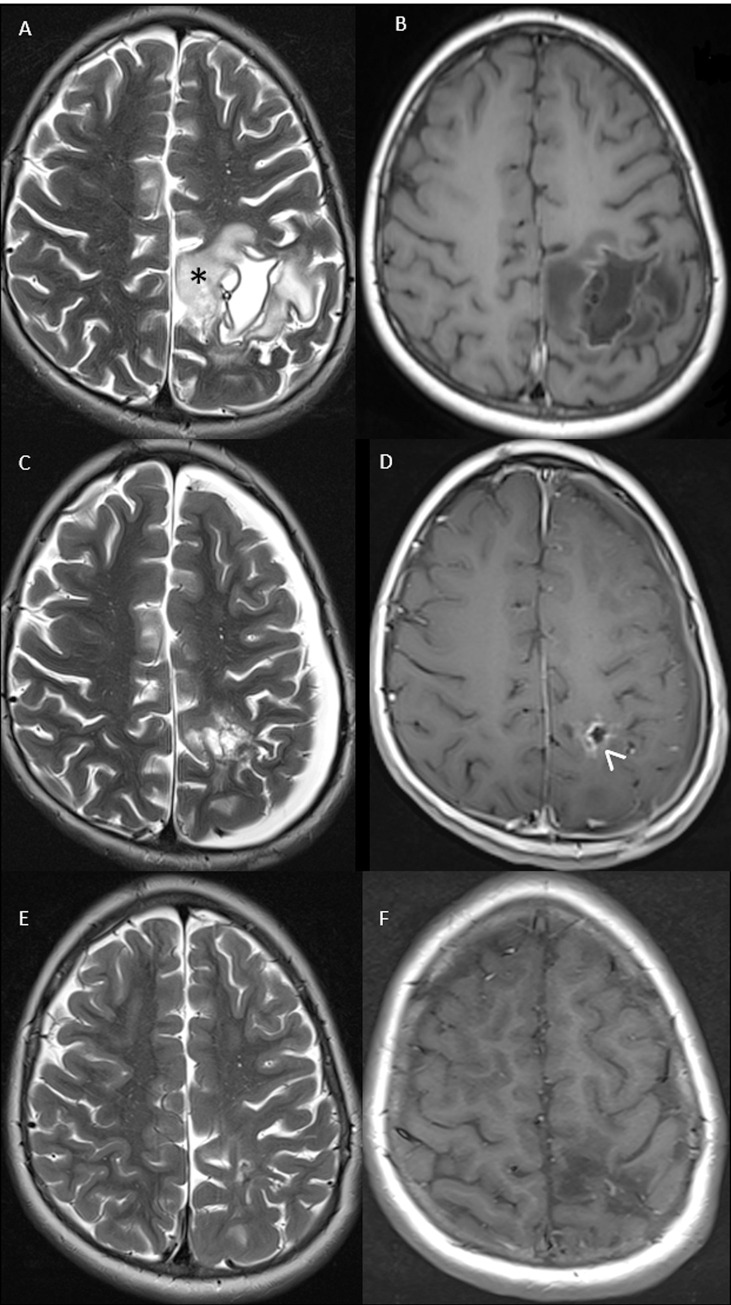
T1 (A, C, E) and T2 (B, D, F) weighted Magnetic Resonance imaging one month after stereotactic cyst drainage (A, B) demonstrated collapse of the cystic cavity (+) and perilesional edema (asterix). Follow-up imaging after 4 weeks of corticosteroid treatment (C, D) demonstrated regression of edema and linear contrast enhancement of the surgical cavity (arrowhead). Long term follow-up (E, F), 12 months after surgery, showed complete disappearance of the lesion.
